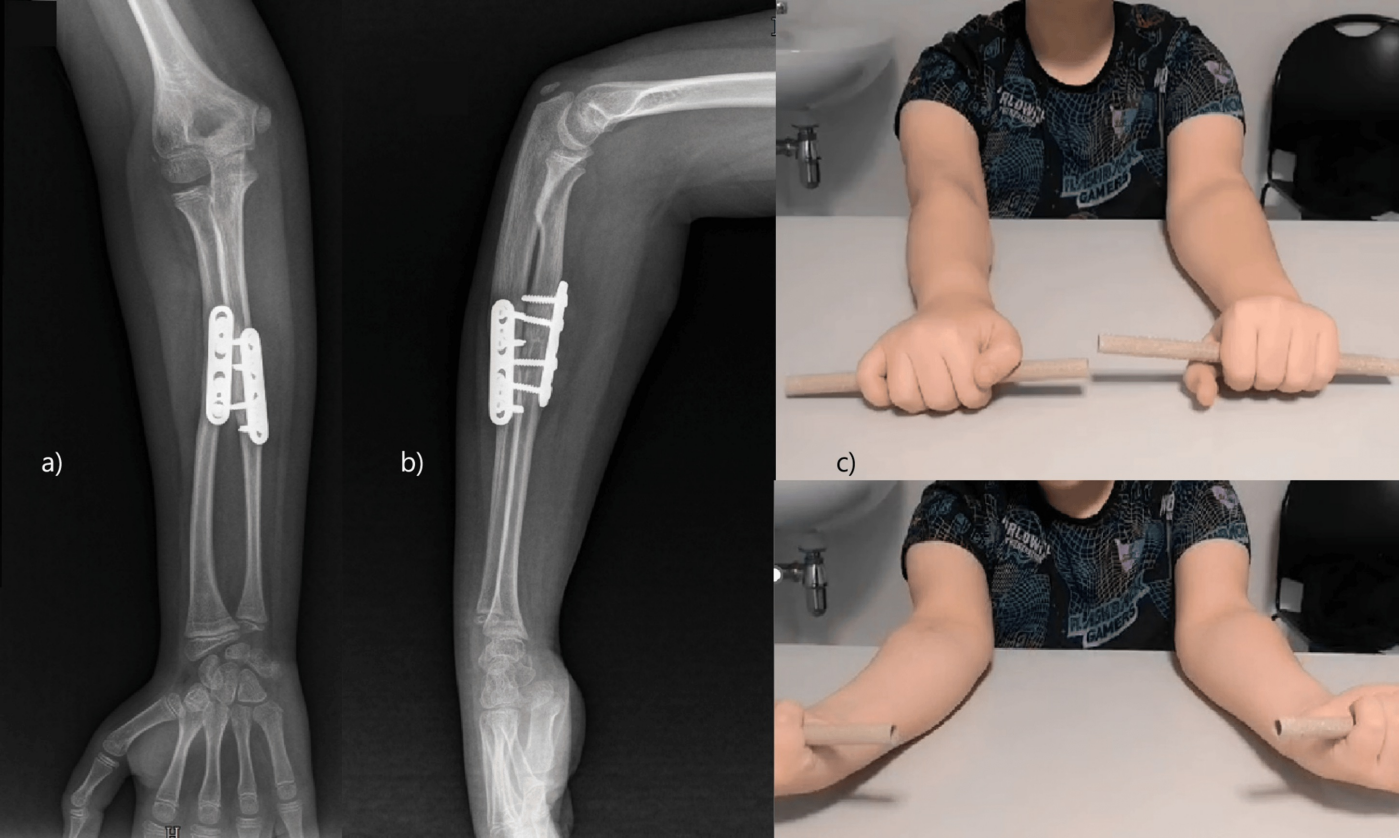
Re-evaluation at one year after surgical procedure a) Anteroposterior view of forearm radiograph; b) side view of forearm radiograph; c) physical examination with pronosupination symmetry.
